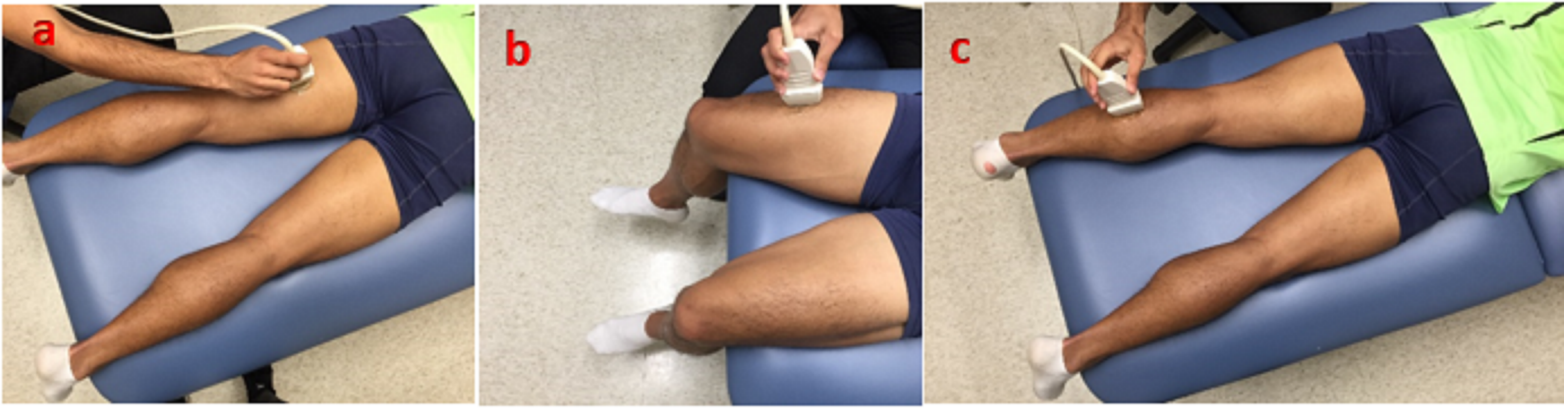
Ultrasound imaging with the transducer at different postures. (A) Posterior thigh muscles (B) anterior thigh muscles (C) calf muscles.
